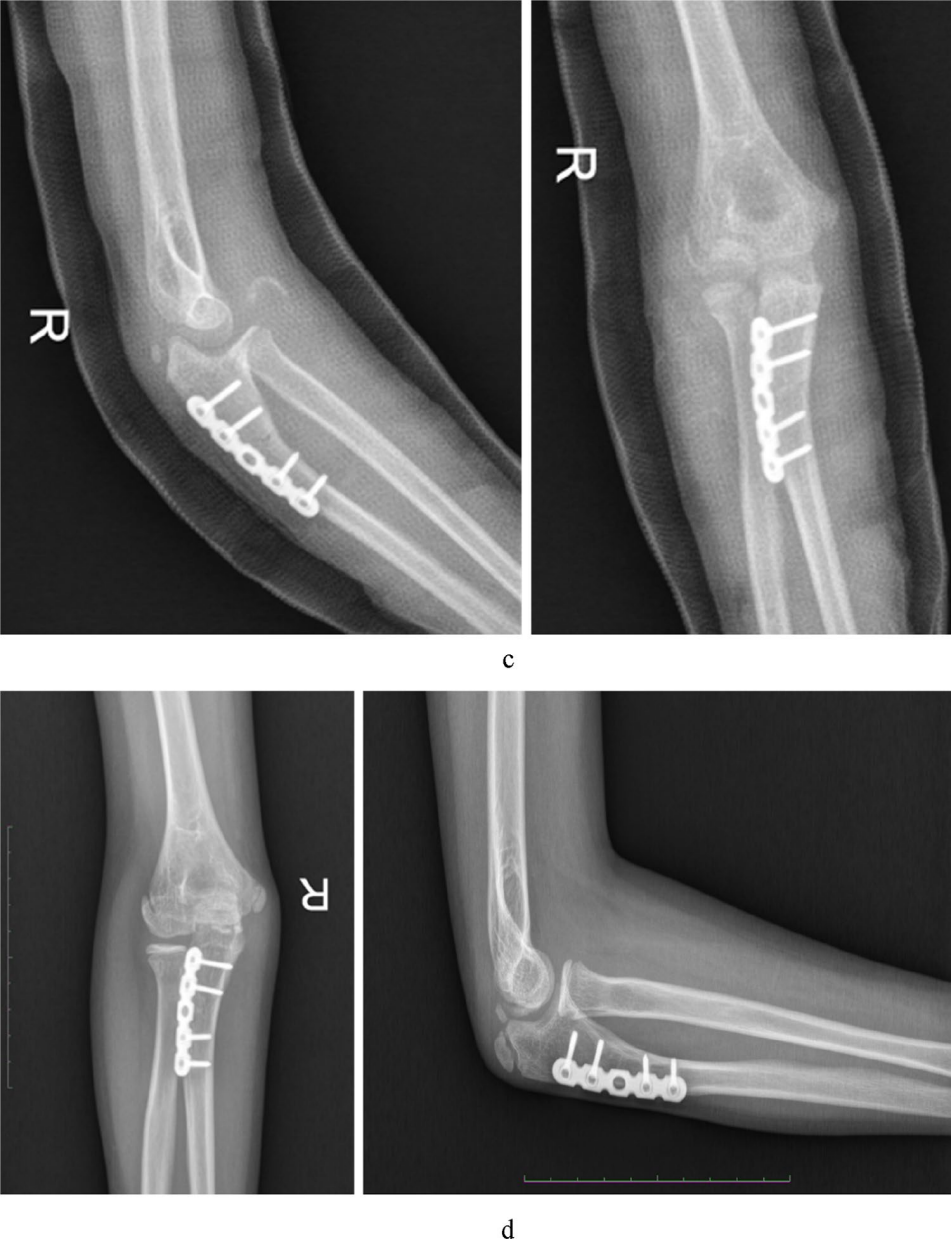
A 9 years old girl (case 4) had fallen from chair. She underwent treatment for a chronic Monteggia fracture after 1 month. (a) Preoperative X-ray. Heterotopic ossification can be seen. (b) Preoperative MRI. Axial T1- and T2-weighted images demonstrated the dislocation of the proximal radioulnar joint; axial and coronal T2-weighted images showed the concave radial notch of the ulna (the arrow); sagittal T2-weighted images displayed the dislocation of the humeroradial joint and the concave radial head (the thin arrow) combined with the folded and tortuousness annual ligament (the gross arrow). (c) Open reduction with ulnar osteotomy was undertaken. (d) The radial head remained concentric (Grade 1) and heterotopic ossification disappeared at 1 years after operation.
